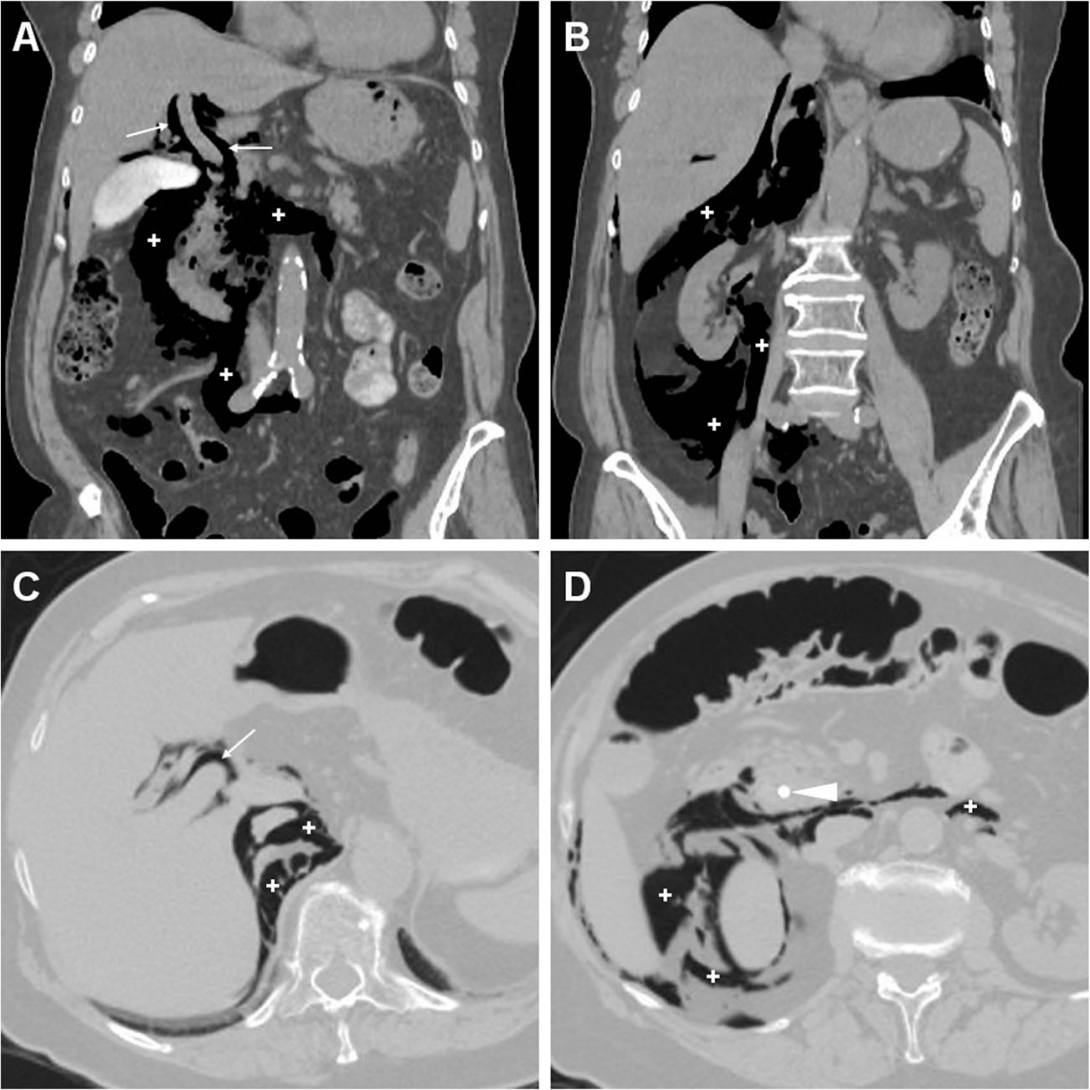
Severe retroperitoneal (type II) DP in an 80-year-old female following ERCP with sphincterotomy, incomplete removal of choledocholithiasis and positioning of a plastic CBD prosthesis (*arrowhead*). Post-procedural unenhanced CT viewed at both soft-tissue (a, b) and bone (c, d) window settings showed extensive retroperitoneal air (+) predominantly located in the right peri- and pararenal spaces, around the descending duodenum and tracking along the inferior vena cava and portal vein (*thin arrows*). Surgical exploration dictated by sepsis failed to detect a perforation site, and the patient recovered fully
